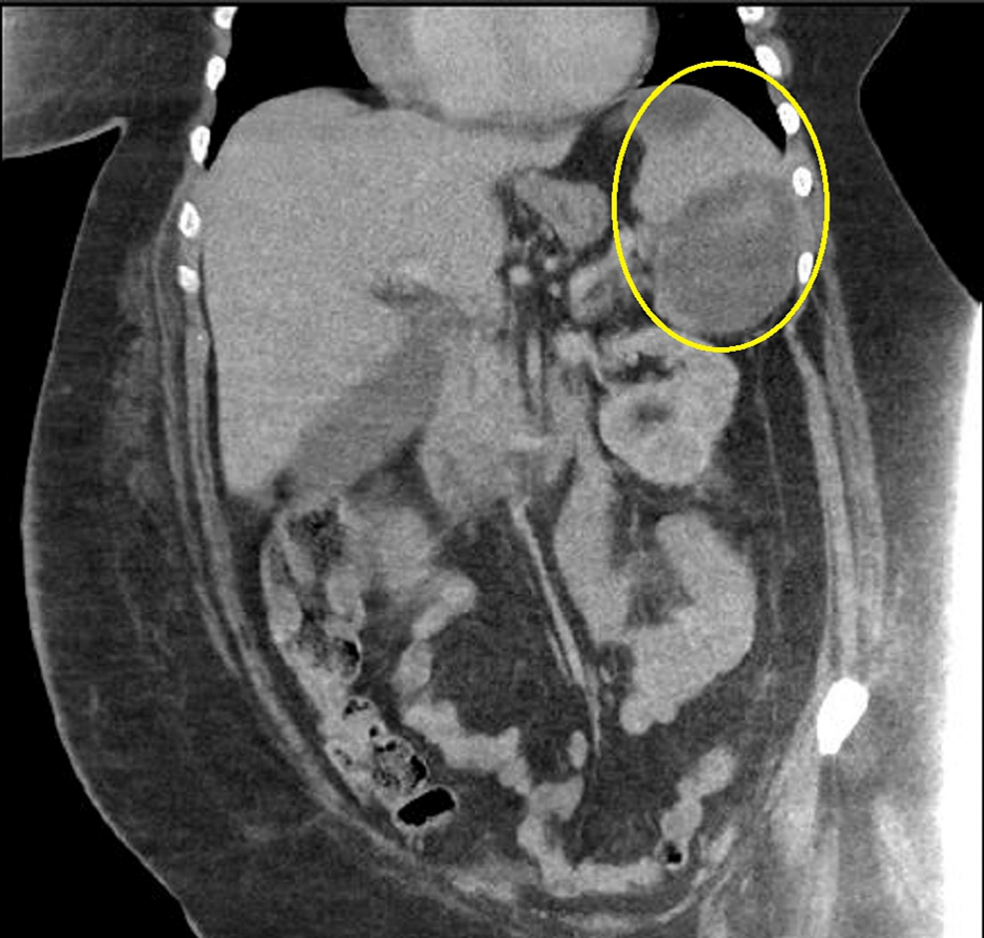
Coronal section of repeat CT scan of the abdomen showing the expansion of splenic lesion and multiple areas of decreased attenuation CT, computed tomography
